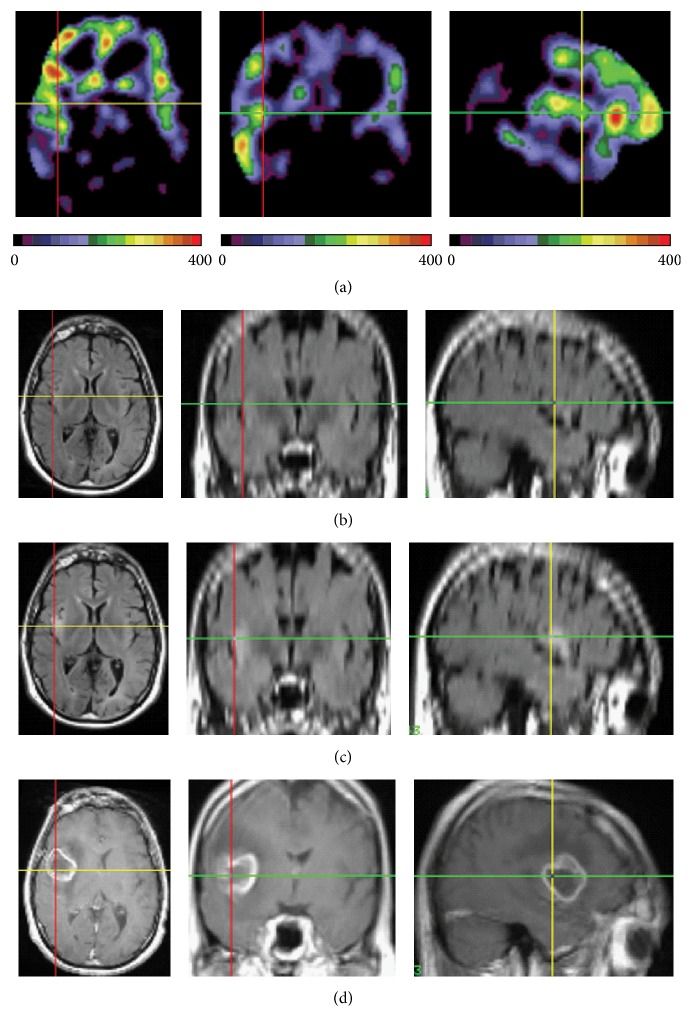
PET and MRI axial (left row), coronal (middle row), and sagittal (right row) images from an individual with familial Alzheimer's disease and GBM (participant #13). (a) AG PET image showing regional asymmetry with higher AG in the right hemisphere. (b) FLAIR MR image at the time of PET study, showing no evidence of abnormality. (c) FLAIR MR image a year later demonstrating first evidence of abnormal signal; (d) MR T1-weighted image with (Gadolinium) contrast enhancement 21 months after PET study, showing GBM.
